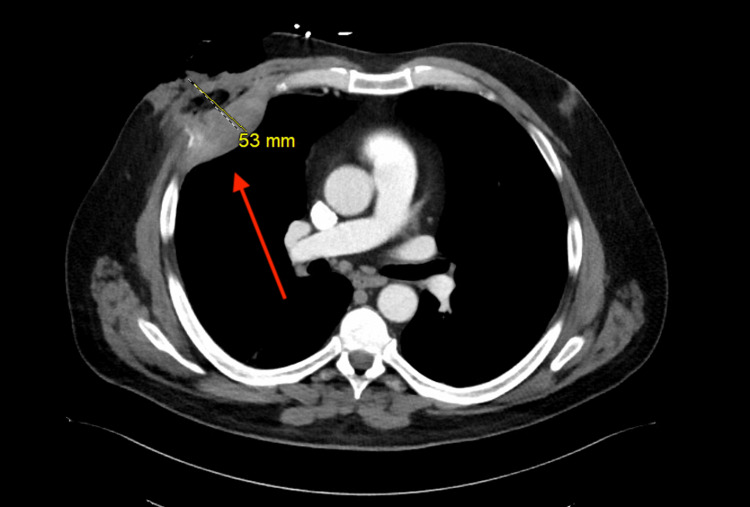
Chest CT scan with IV contrast. Contrast-enhanced axial CT image of the chest demonstrating a heterogeneous soft tissue mass involving the right anterior chest wall, with extension through the intercostal space (red arrow), measuring approximately 53 mm in maximal dimension.
